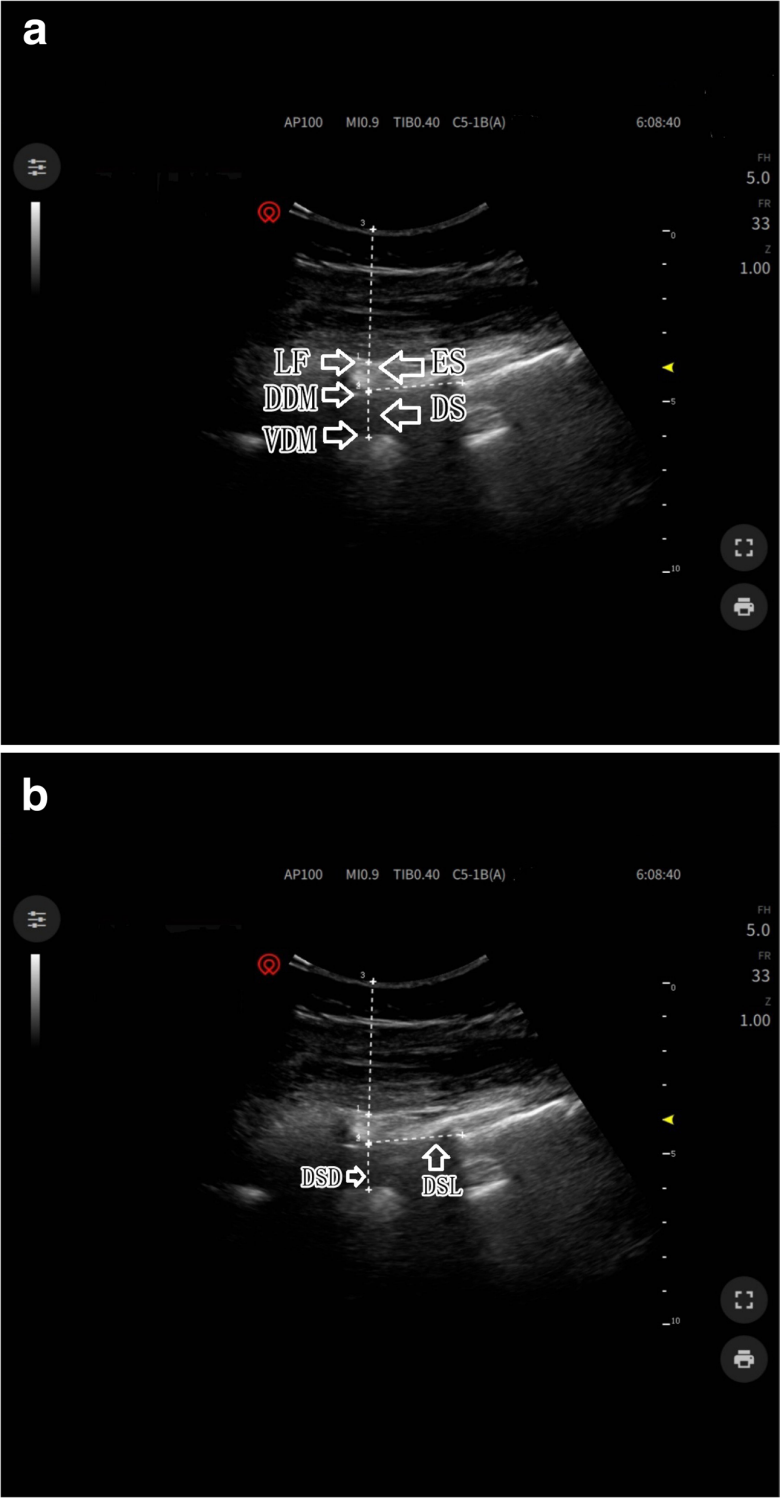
Measurement of the anatomical dimensions of the dural sac by ultrasound. A LF = ligamentum flavum, DDM = dorsal dura mater, VDM = ventral dura mater, ES = epidural space, DS = dural sac. B DSD = dural sac diameter, DSL = dural sac length
